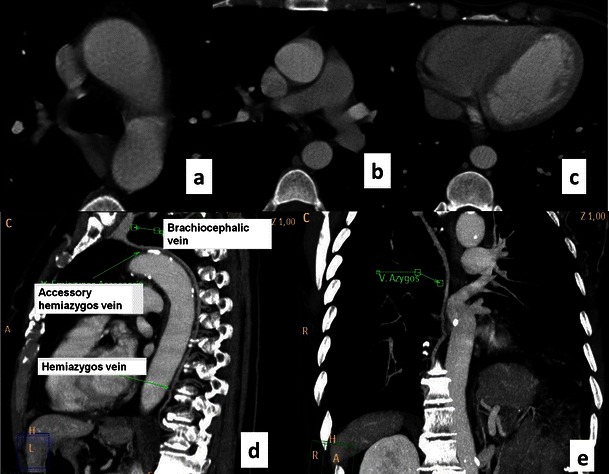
CT of the normal azygos venous system. Contrast-enhanced CT axial images show the normal appearance of the azygos vein arching from the right paraspinal space to the superior vena cava (a). The azygos vein runs longitudinally on the right side of the thoracic vertebral bodies (a). The hemiazygos vein runs on the left side of the spine (b) until anastomosis with the azygos vein on T8-9 as shown in the sagittal and coronal view (c–d)
